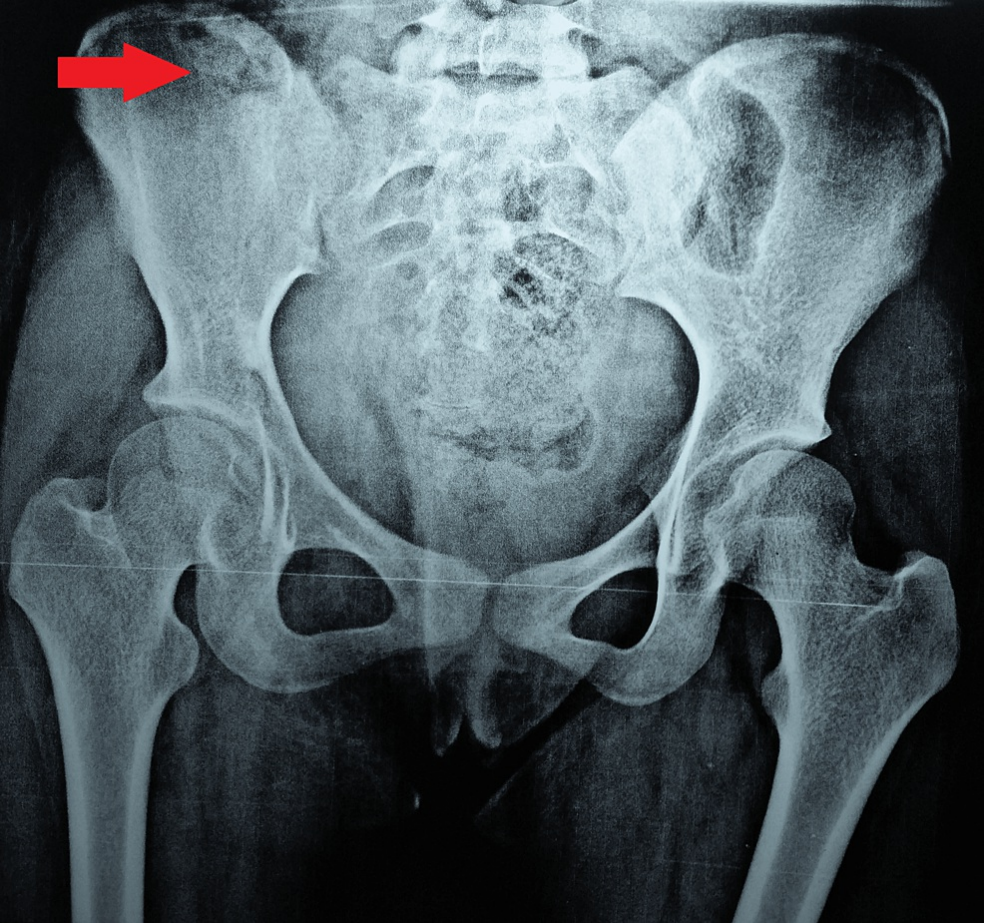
Plain radiograph of the pelvis (AP view) AP: anteroposterior
